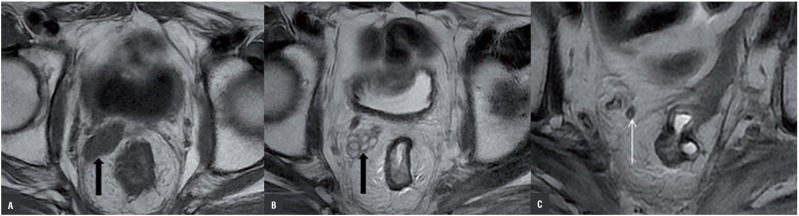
Left SV agenesis of a 63 years old male patient who had colon ca. Axial (a) T1 weighted MR image, axial; (b) T2 weighted MR images show left SV absence. Right SV normal (thick black arrow). Axial (c) T2 weighted MR image show; SV absence was associated with left VD absence. Right VD normal (white thin arrow). Postoperative changes at the anastomosis line of the rectosigmoid junction.
